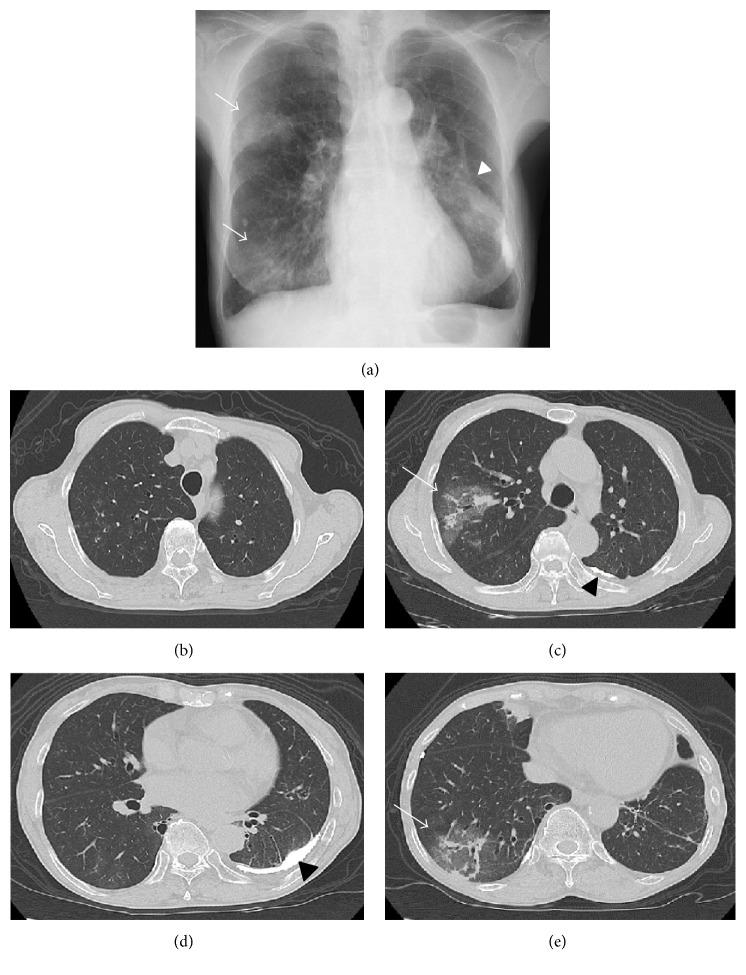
Findings on chest radiography and computed tomography. (a) Chest radiograph showing reticular shadows in the right middle and lower lung fields (arrow). Calcified lesions were seen in the left lung field (arrowhead). (b–e) Chest computed tomography (CT) showing segmental ground glass shadows in the upper and lower lobes of the right lung (arrow). The left pleura shows thickening with calcification (arrowhead).
